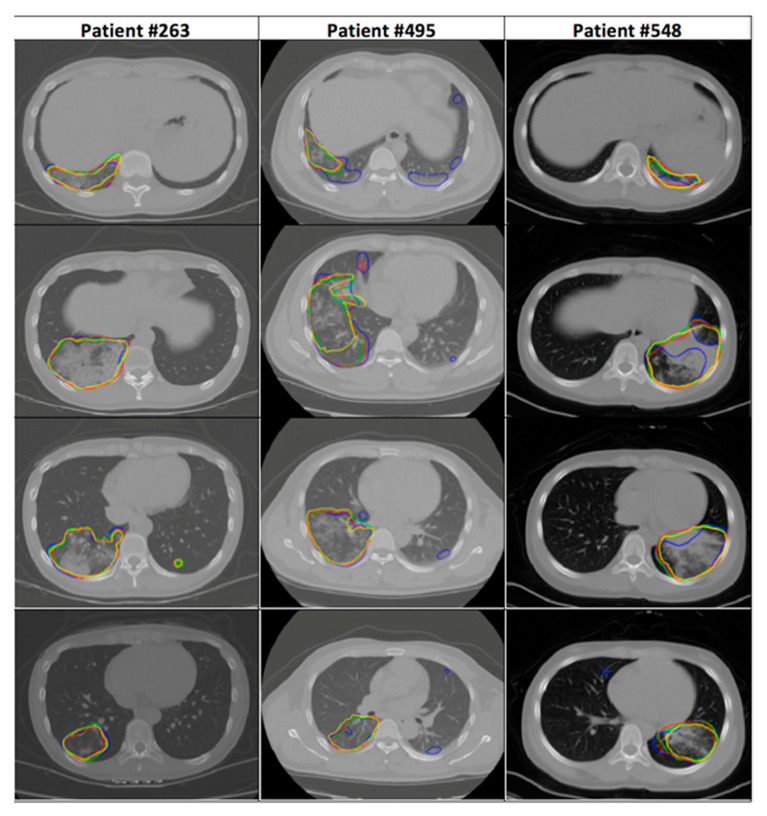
CT images showing parenchyma with visible subregions (yellow for the gold standard, red for C-ENET, green for ENET, and blue for ERFNET,) infected with COVID-19 disease.
